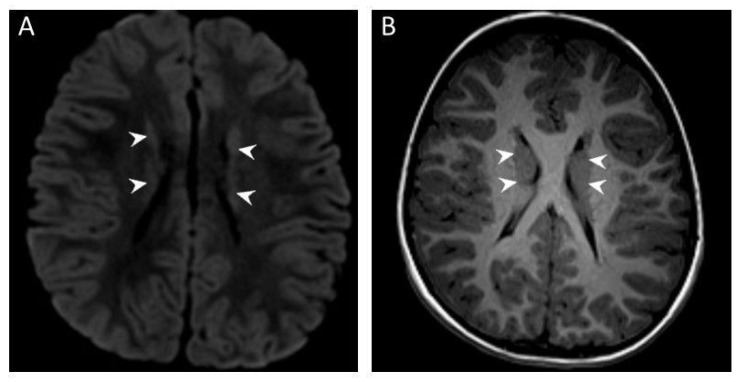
Brain MRI in our patient showing bilateral periventricular nodular heterotopia (arrowheads indicate ectopic gray matter nodules): T2 FLAIR (A) and T1 (B) axial brain MRI image.
